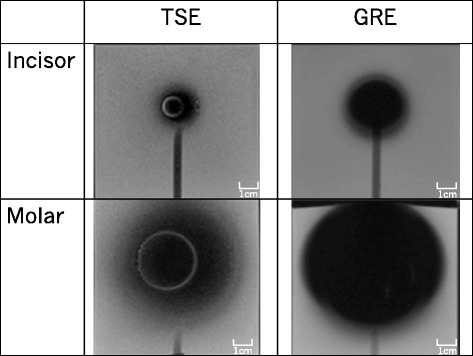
Magnetic resonance images of incisor and molar brackets taken with turbo spin echo (TSE) and gradient echo (GRE) sequences
